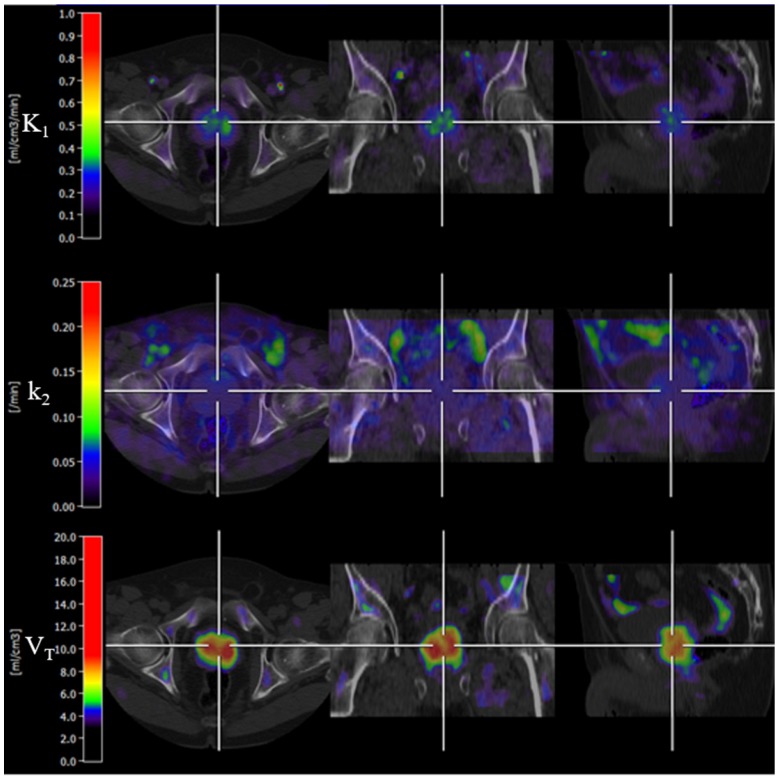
An example of parametric images of K_1_, k_2_ and V_T_ (Patient 2). V_T_ parametric image showed bilateral signal uptake in the prostate gland. The first, second and third rows demonstrate trans-axial, coronal and sagittal PET/CT fused images, respectively.
